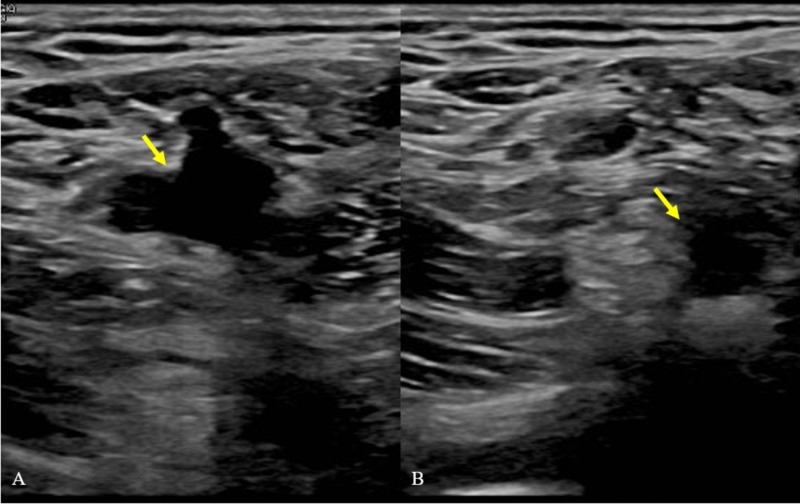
Duplex venous ultrasound of the left lower femoral vein (A) Representative image of the non-compressed femoral vein. The yellow arrow shows an uncompressed patent femoral vein. (B) Lack of complete collapse upon compression is indicative of venous thromboembolism. The yellow arrow shows the partially compressed femoral vein.
